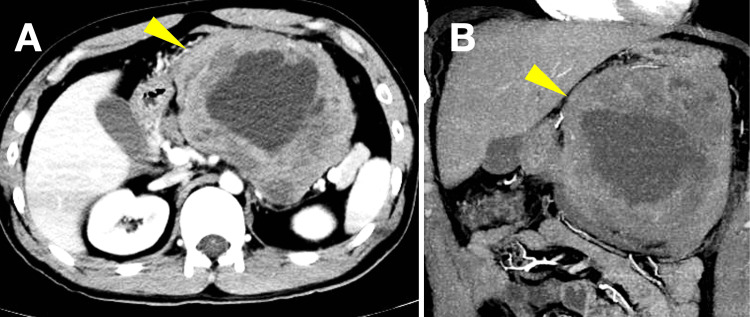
Preoperative contrast-enhanced CT (A) Axial view demonstrating a large heterogeneous mass (arrowhead) arising from the stomach with internal hemorrhage and areas of necrosis. (B) Coronal view showing the extent of the tumor (arrowhead) and associated hemoperitoneum with free fluid in the peritoneal cavity CT: computed tomography
